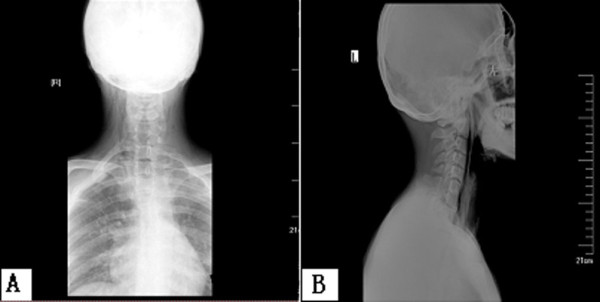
The anteroposterior (a) and lateral view X-ray (b) of the neck. It reveals subcutaneous emphysema but no foreign body was observed in the soft tissues.
